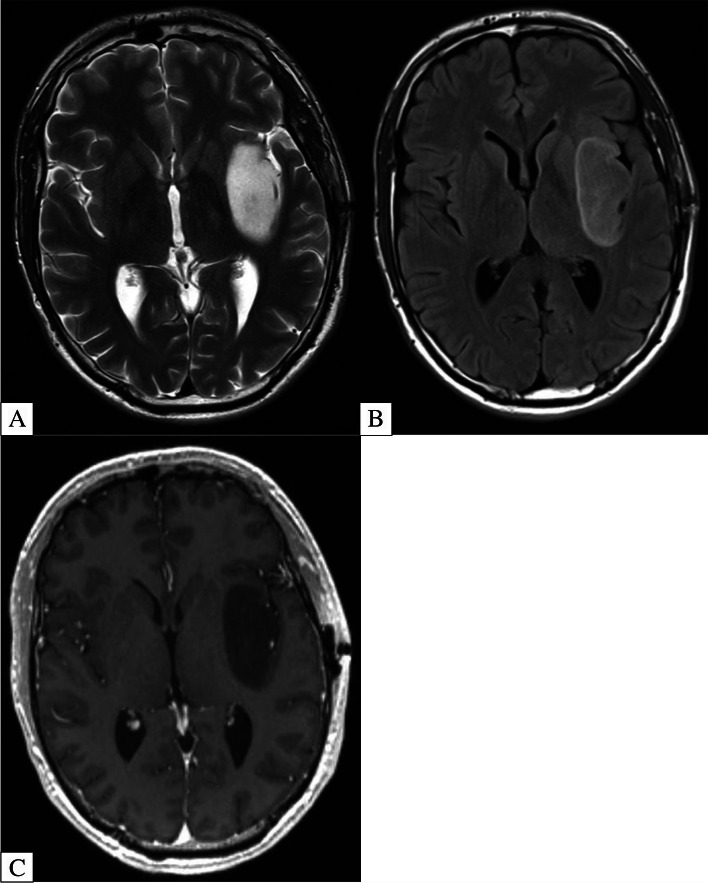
A-C 26-Year-Old Patient with Biopsy Proven IDH-Mutant Astrocytoma Showing the T2/FLAIR Mismatch Sign. Axial T2-Weighted MRI (A) shows a homogenously T2 hyperintense lesion centered within the left insula. Axial FLAIR MRI (B) shows the lesion becomes relatively hypointense with peripheral hyperintense rim due to incomplete suppression. Axial T1-Weighted Post Contrast MRI (C) shows no substantial enhancement within the lesion
